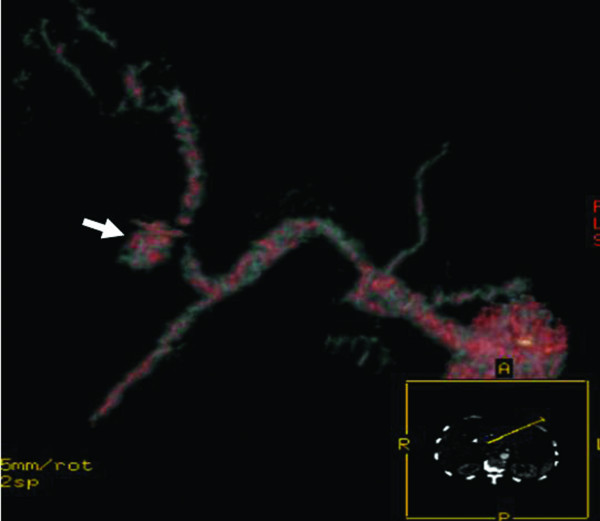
Reconstructive CT image showing a saccular mass protruding from the proper hepatic artery.
